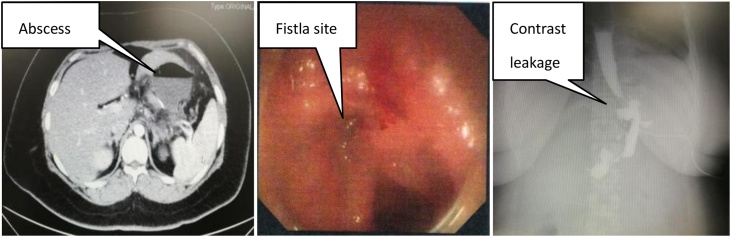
The CT, gastroscopy and esophagography revealed that the presence of anastomotic leakage and abscess beside the stomach.
